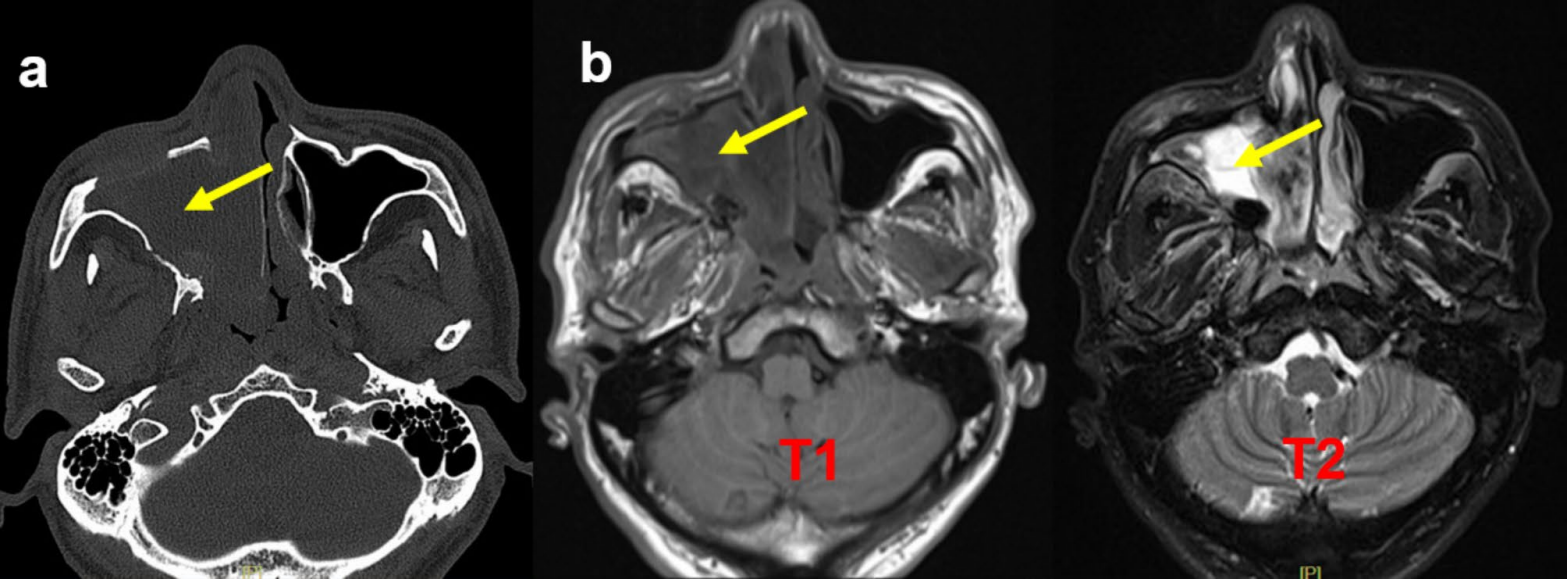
CT scan of the paranasal sinus (2021-04-15) (a). Enhanced MRI Scan of the paranasal sinus (2021-04-20) (b). The mass in the right maxillary sinus had by the sinus (arrow)
